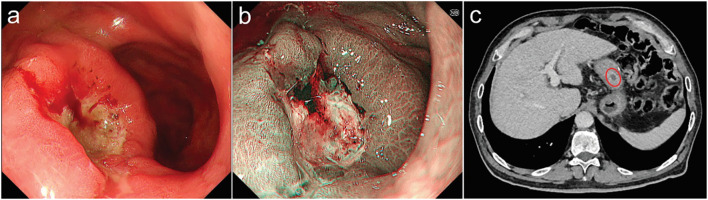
Endoscopic images one year after SASI surgery (July 2025). An ulcerative lesion is visible on the posterior wall of the gastric body. (a) Gastroscopic view in standard white-light mode; (b) Gastroscopic view in narrow-band imaging (NBI) mode; (c) CT images one year after SASI surgery showing localized enhancing wall thickening around the ulcer site (indicated by red circle).
